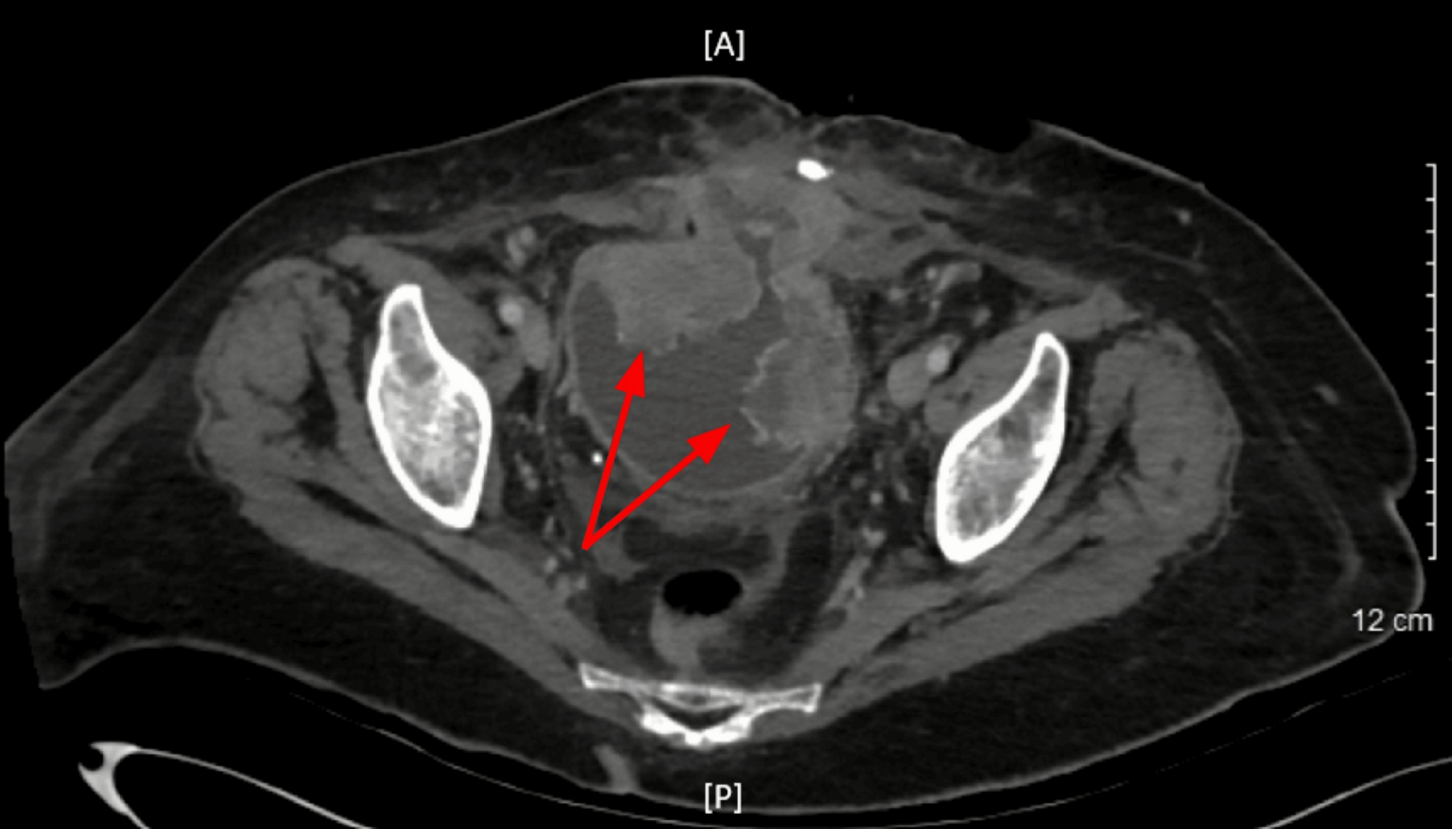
Axial computed tomography scan of the abdomen and pelvis The red arrows show an infiltrative mass involving the anterior and left lateral bladder walls extending into the herniated portion of the urinary bladder. This mass was later diagnosed as high-grade urothelial carcinoma. A: anterior; P: posterior
